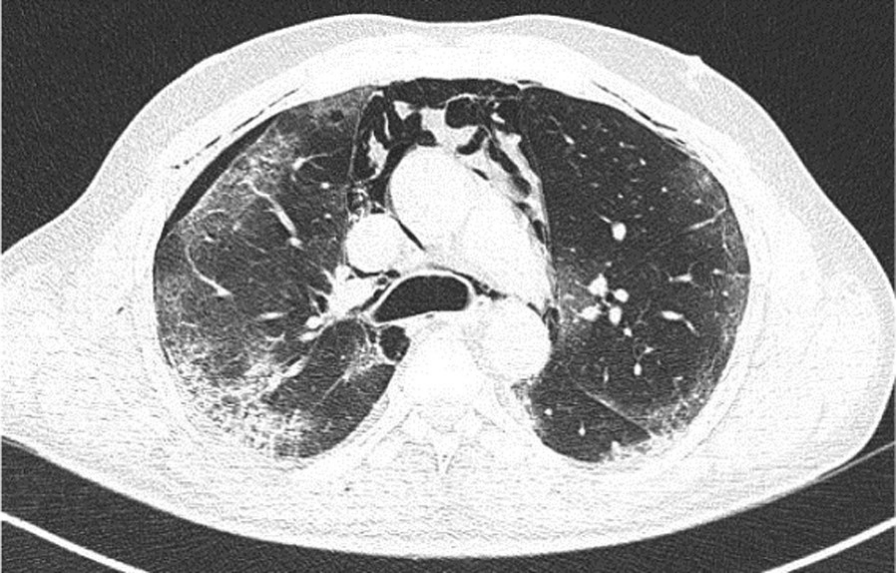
CT scan chest showing mediastinal and surgical emphysema
